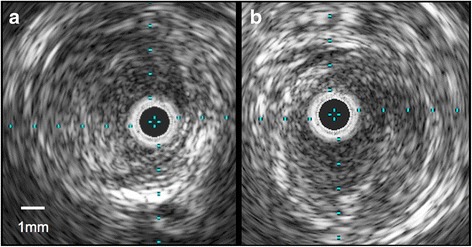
Intravascular ultrasound image. IVUS imaging in the external iliac lesion showing the wire passing through the true lumen and the mixed plaque with no calcification. (a proximal site of the left external iliac artery, (b) distal site of the left external iliac artery)
